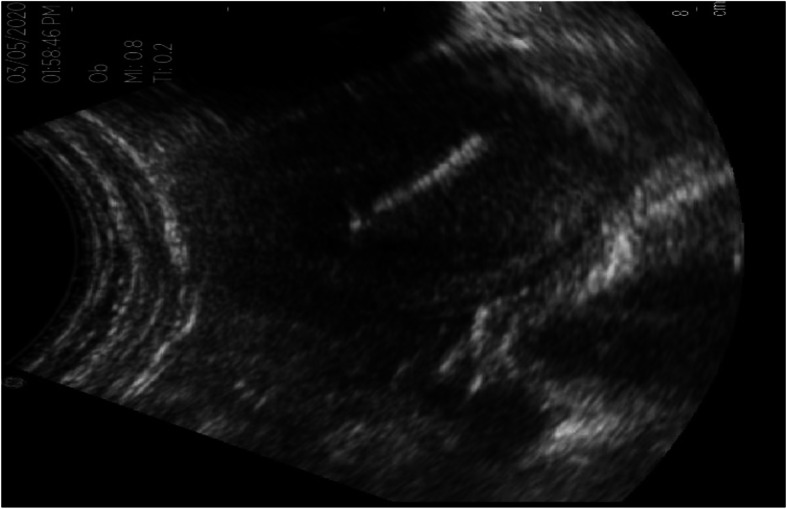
Transabdominal ultrasonic image of the intrauterine contraceptive device (copper-T 380A IUCD). Ultrasound image showed a centrally located copper-T 380A IUCD in the endometrial cavity
